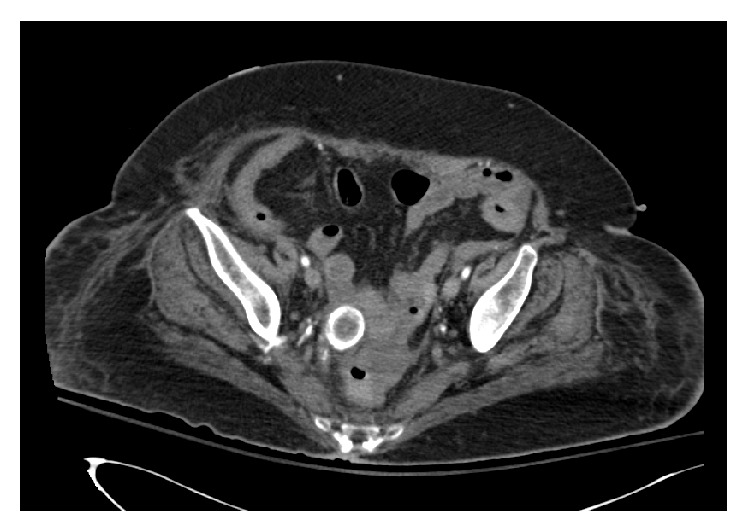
Abdomen and pelvis CT one month prior to obstructive presentation revealing large gallstone resting in the pelvis outside of the bowel lumen.
